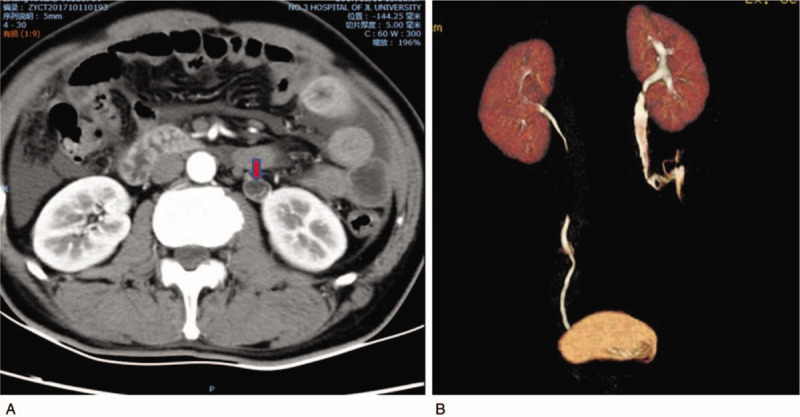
CT angiogram showing thicker proximal ureter while the distal part is not discernible; the ureter cannot be traced distally in CT. Red arrow indicates the necrotic proximal ureter with edema.
